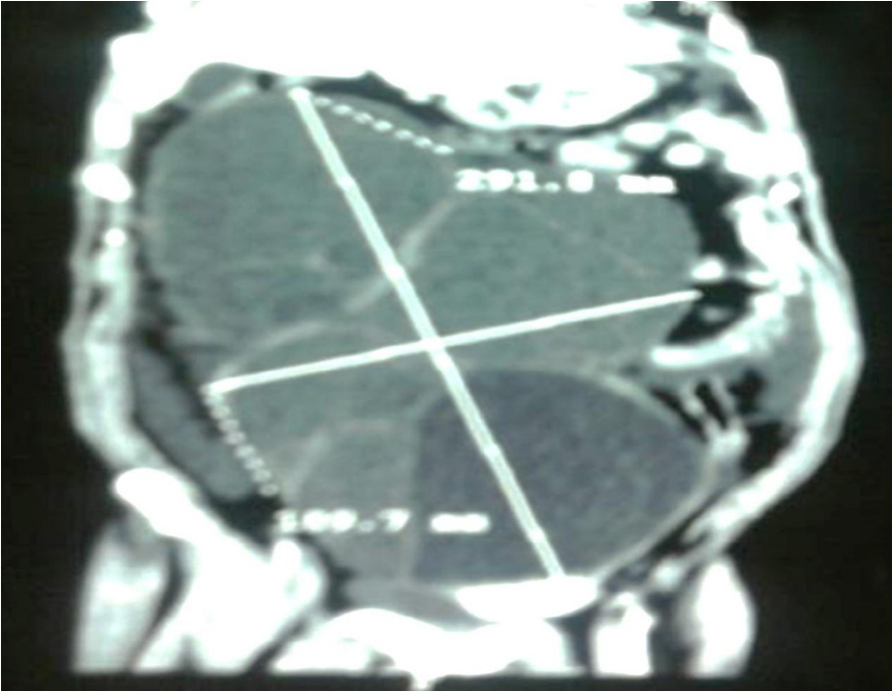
Computed tomography scan showing a huge tumor with tissue and cystic components.
